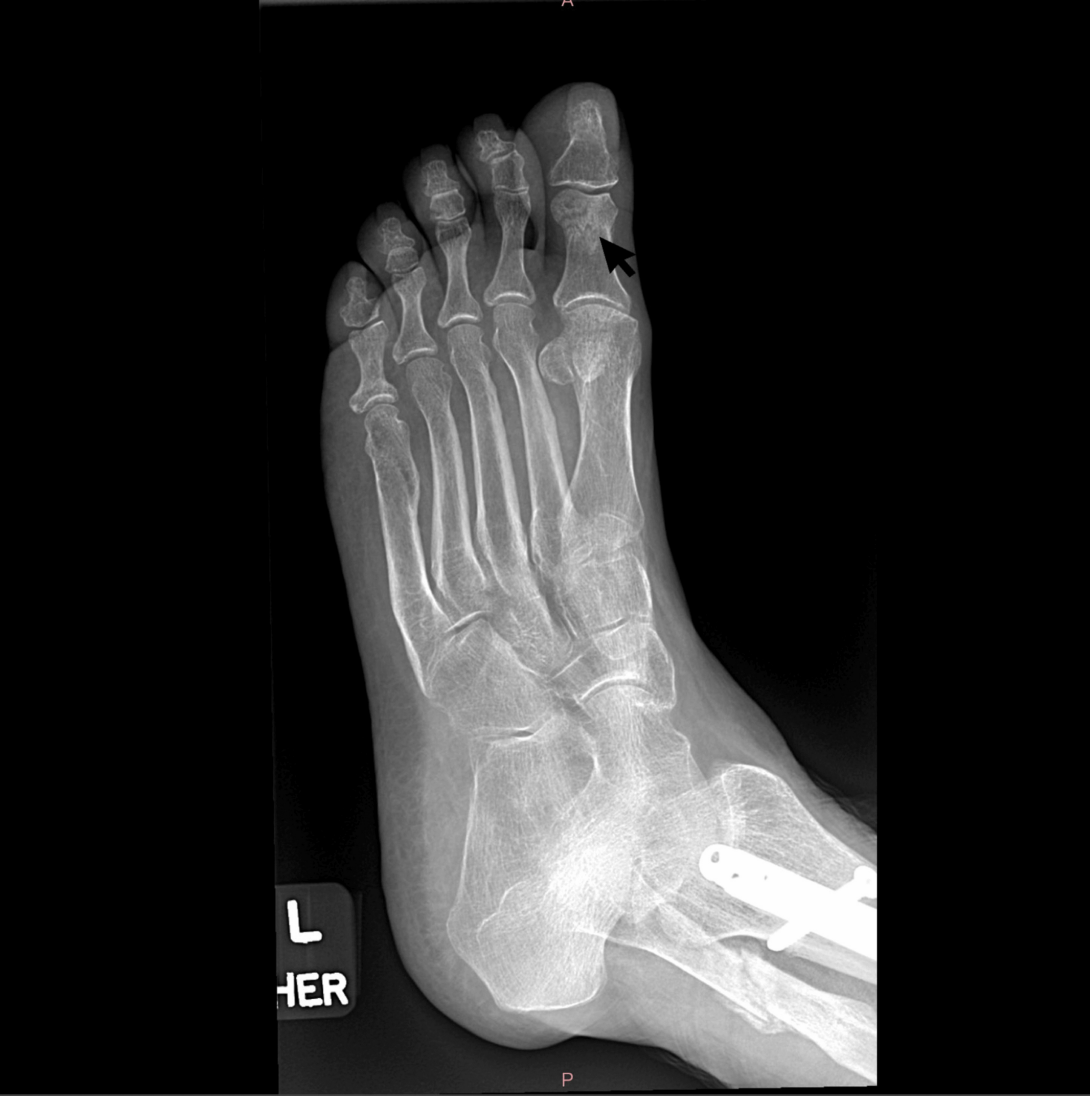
X-ray imaging showcasing fracture of the proximal phalanx of the left great toe
